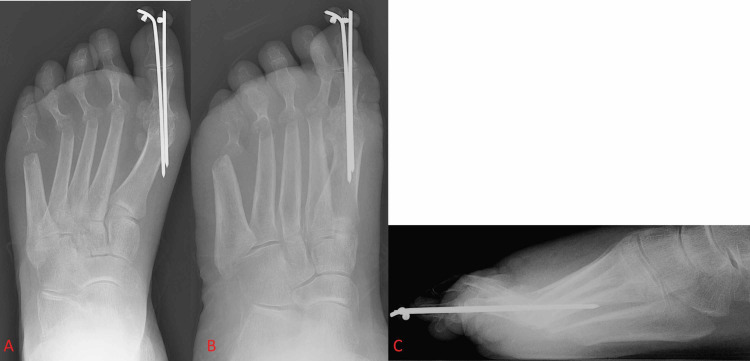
Foot X-ray shows Kirschner wire fixation of the first metatarsophalangeal joint. Figure 2A and Figure 2B show anterior-posterior and oblique views of the foot, respectively. Figure 2C shows a lateral view of the foot.
